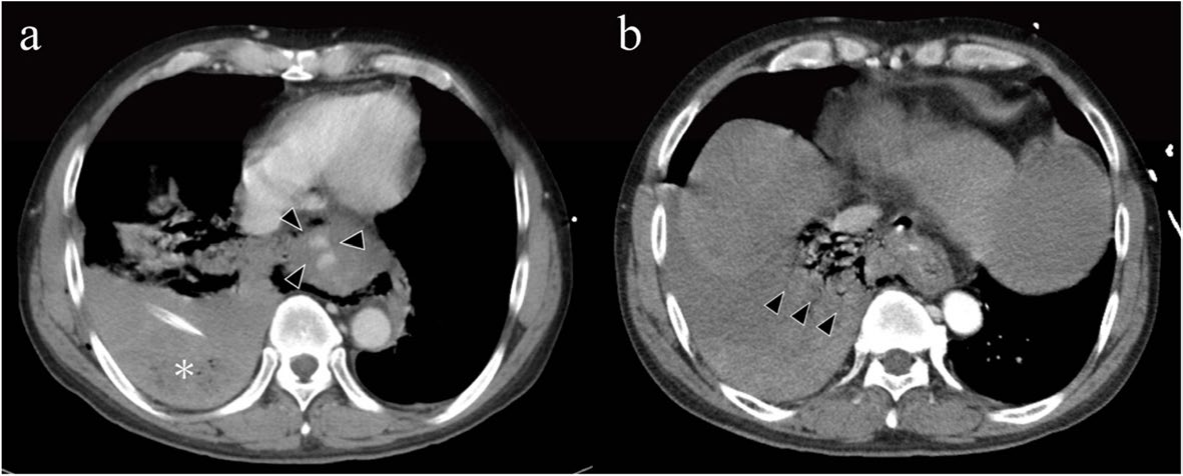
a Computed tomography shows pleural effusion in the right thoracic cavity (asterisk) and contrast leak into the esophagus (triangle). b Disruption of the esophageal wall in the right thoracic cavity (triangle)
